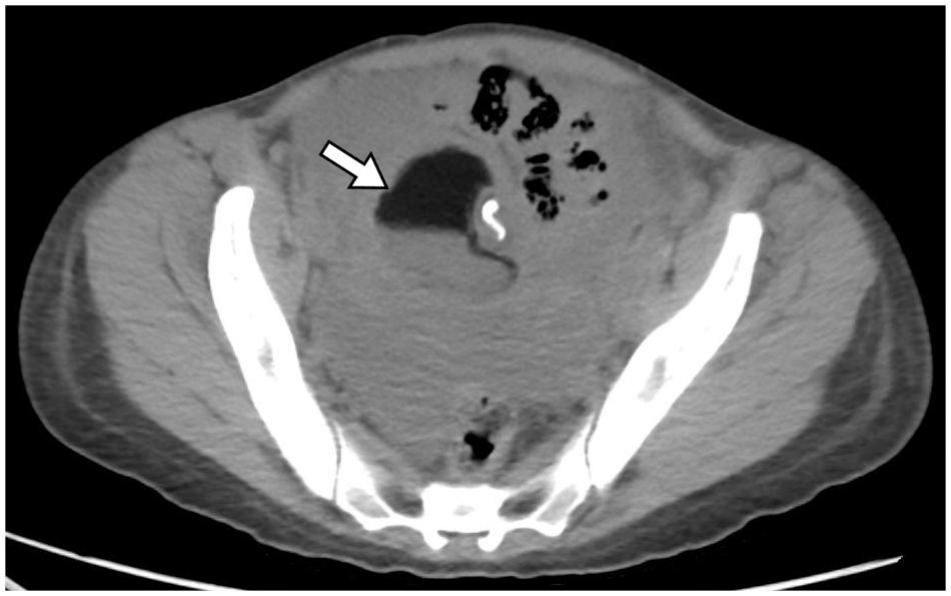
Axial CT section at the level of the pelvis shows a mass in the right adnexa with internal fat, fluid, and calcifications, consistent with a dermoid cyst. There is an internal fat-fluid level. There is also hemorrhagic fluid surrounding the cyst in the pelvis.
